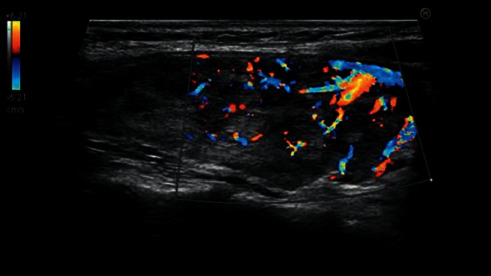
Color Doppler ultrasound grading of hyperthyroidism level 1.
